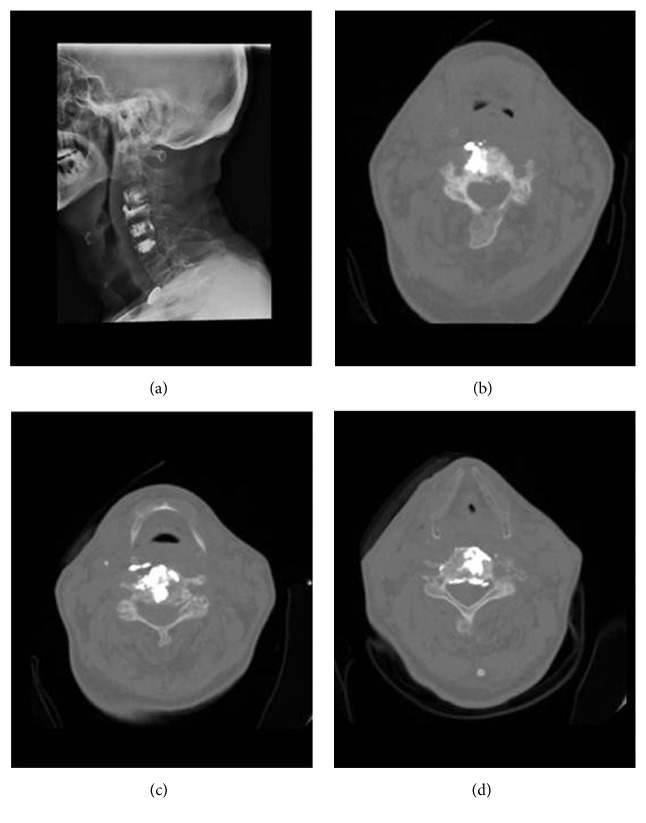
(a) CT image of one patient after PVP was performed in 4 cervical spines. Cement leakage was observed at the right side of (b) C3 and (c) C4 and in the spinal canal of (d) C5.
